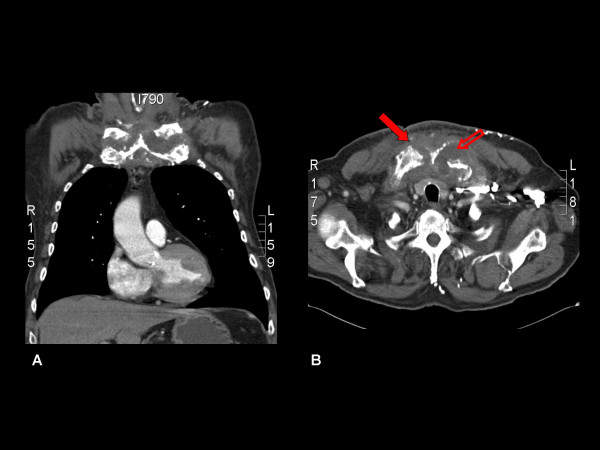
Computed tomography scans demonstrating the destruction of the sternoclavicular joints bilaterally (solid arrow), with a large effusion of the left sternoclavicular joint (hollow arrow). (a) Coronal image; (b) sagittal image.
